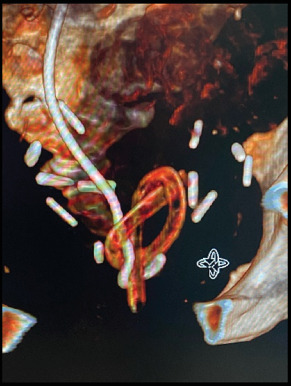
CT 3D reconstructed images of catheter/stent knot.
